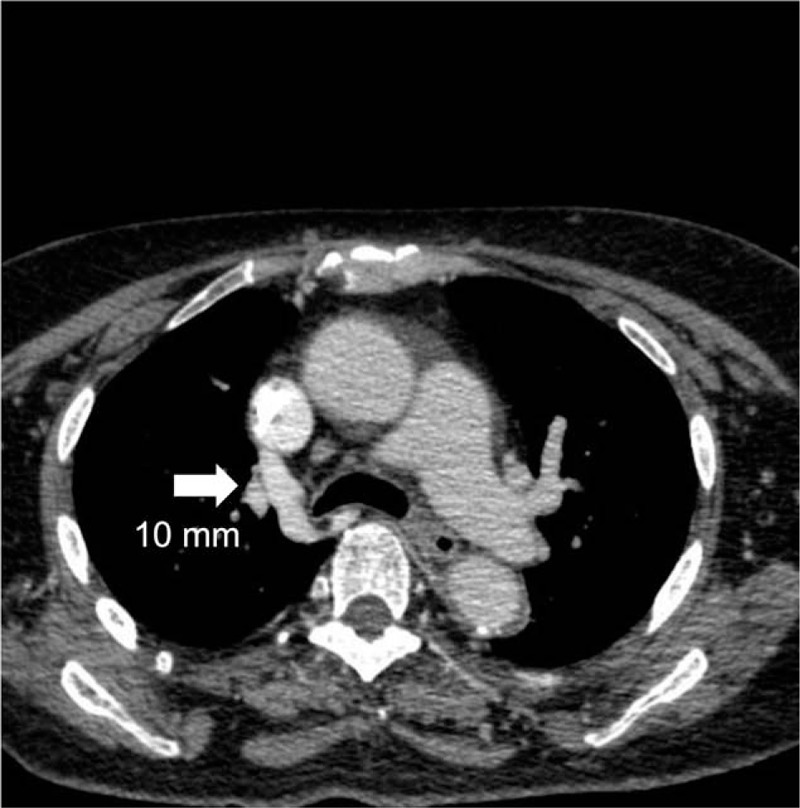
CT scan of azygous vein. The azygos vein was thickened with ostial size of 10 mm (arrow).
